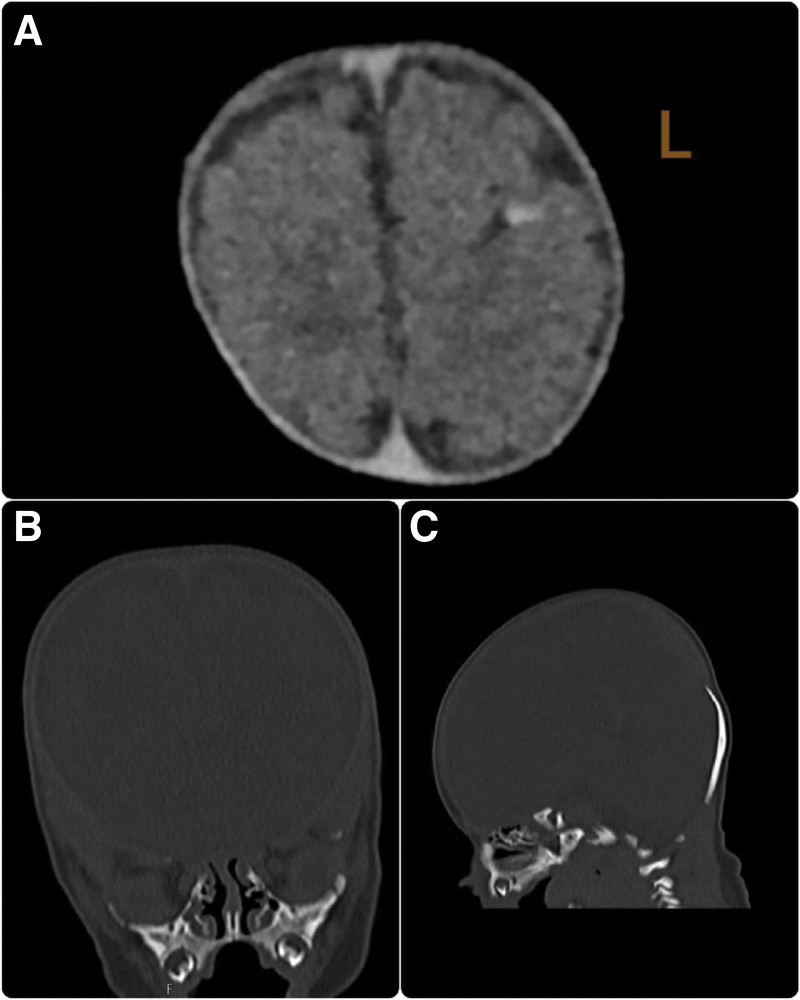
(A) Axial CT scan without IV contrast of the brain window showed foci of parenchymal hemorrhage at the left frontal lobe. (B) Coronal CT scan without IV contrast showed normal facial bones with absent frontal and parietal bones. (C) Coronal CT scan without IV contrast showed absent all calvarium bones with preservation of the occipital bone.
